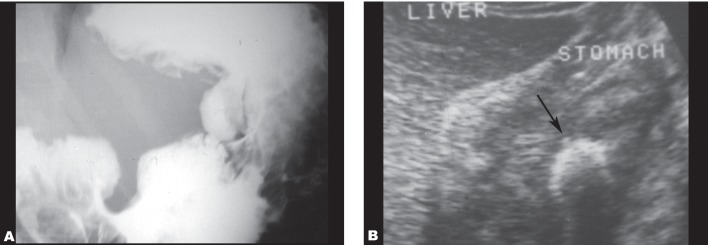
Malignant gastric ulcer in a 55-year-old man.( (A) Shows barium meal in this patient. (B) Abdominal sonography shows thickening of the gastric wall associated with a fixed echogenic area (arrow in B) probably representing the ulcer crater.)
